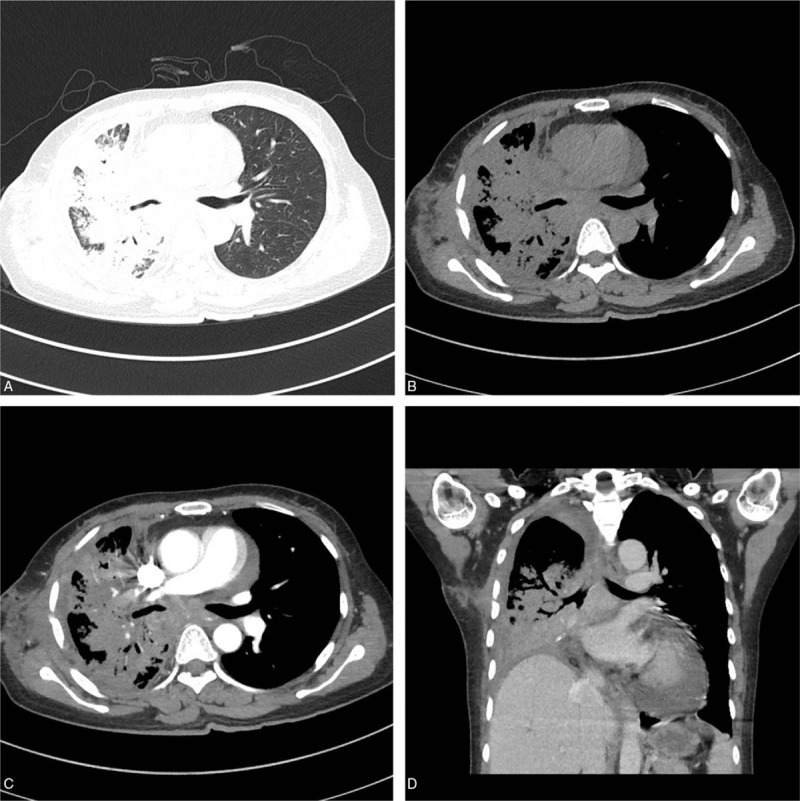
Female, 54 years old, recurrent cough and expectoration for 2 months (removed the right middle and lower lung lobes due to suspected central pulmonary cancer). CT shows post-operation changes of the right middle and lower lung lobes. Multiple consolidation and atelectasis happen in the right upper lung with stenosis of the right main bronchus. There are multiple enlarged lymph nodes in the right hilum and mediastinum with a little fluid in the right pleural cavity and thickening of the right pleura (A–D).
